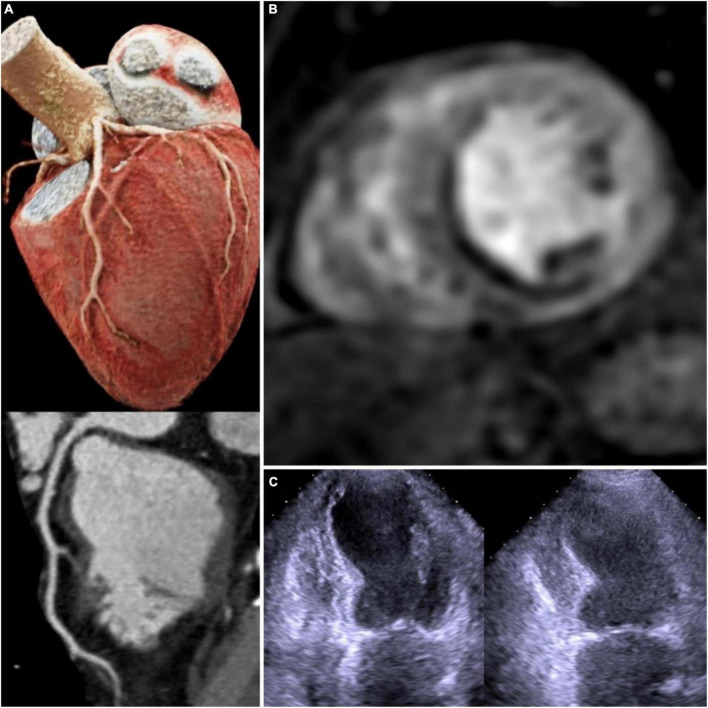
Multimodality imaging in chronic coronary syndrome: 3D CT coronary angiography with left anterior descendent artery curved-MPR–no lesions (A); stress CMR with adenosine—subendocardial infero-septal perfusion deficit (B); dobutamine stress echocardiography—apical inferior wall hypokinesia (C). 3D CT, 3 dimensional computed tomography; MPR, multiplanar reformation; CMR, cardiovascular magnetic resonance.
